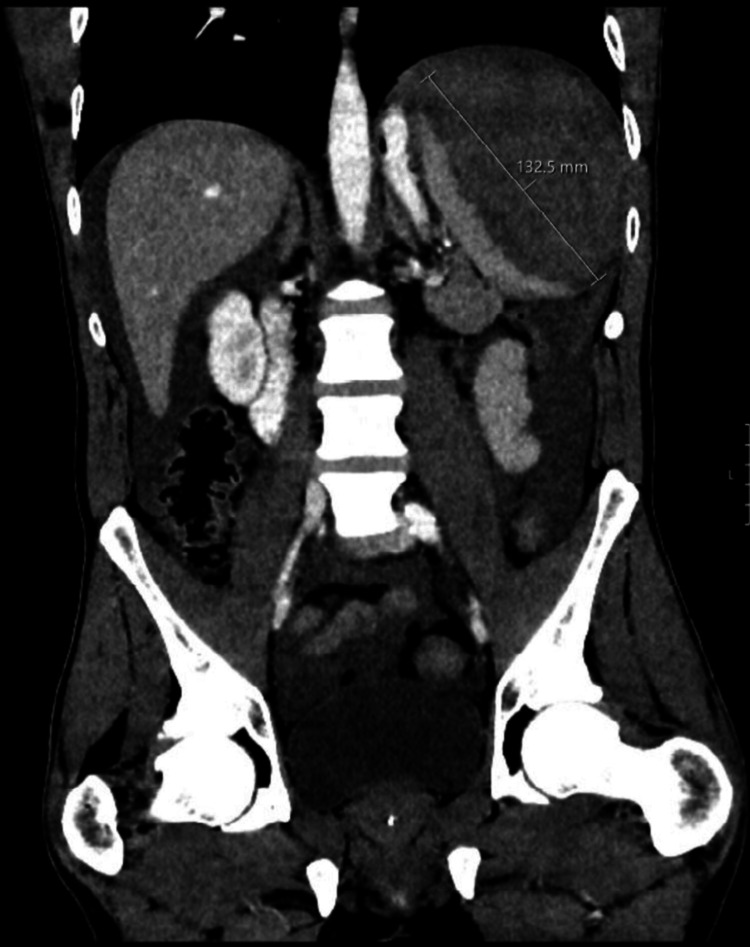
A contrast-enhanced computed tomography scan of the abdomen, revealing a 13.25cm splenic hematoma.
